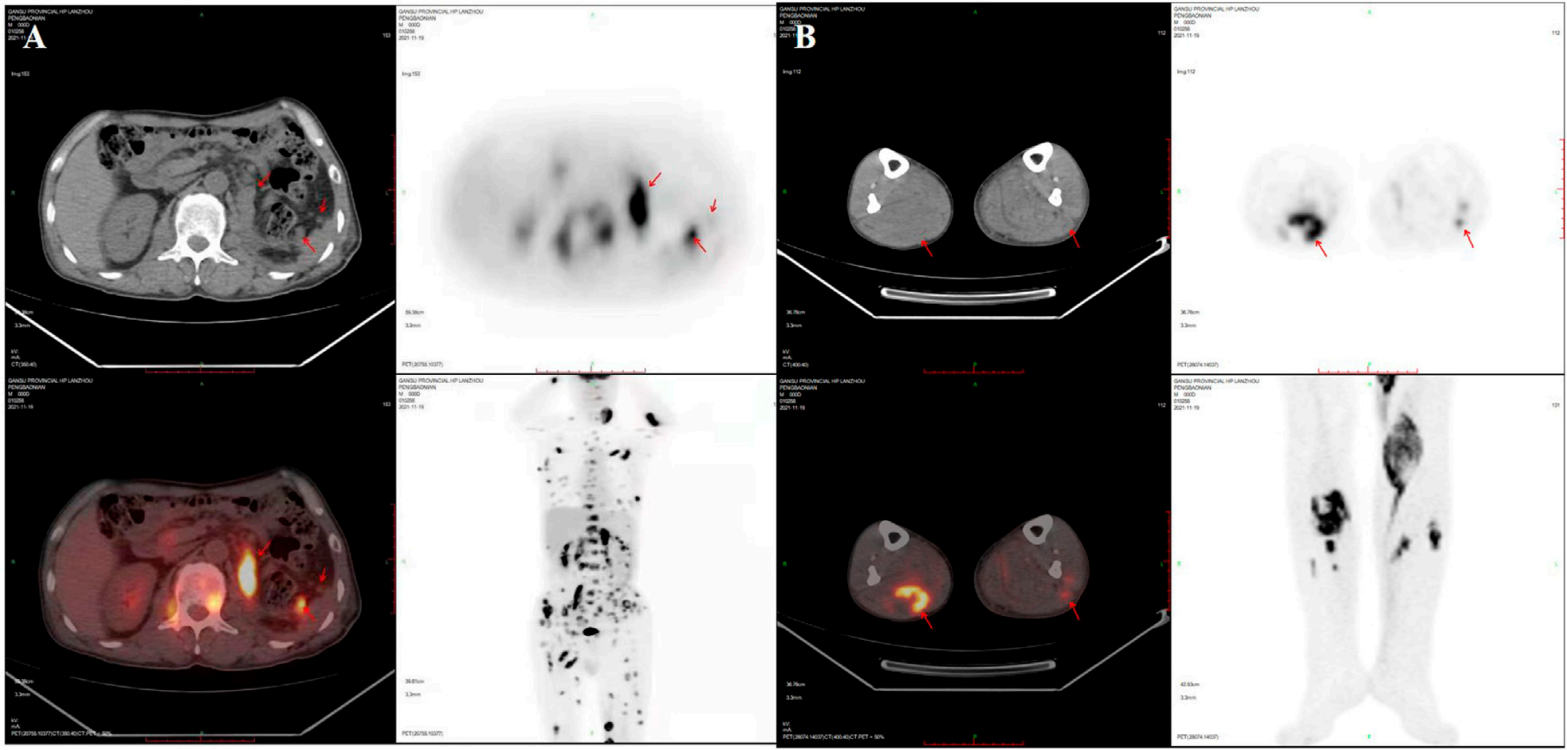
PET-CT scan showed the patient developed multiple nodules of metastasis in the left renal region, extensive systemic muscle, bone, lymph node, liver and bilateral lung metastases. (A) Multiple nodules of metastasis in the left renal region, extensive systemic muscle, bone, lymph node, liver and bilateral lung metastases. (B) Multiple metastases are seen in bilateral lower limb muscles. SUVmax, maximum of standardized uptake value.
